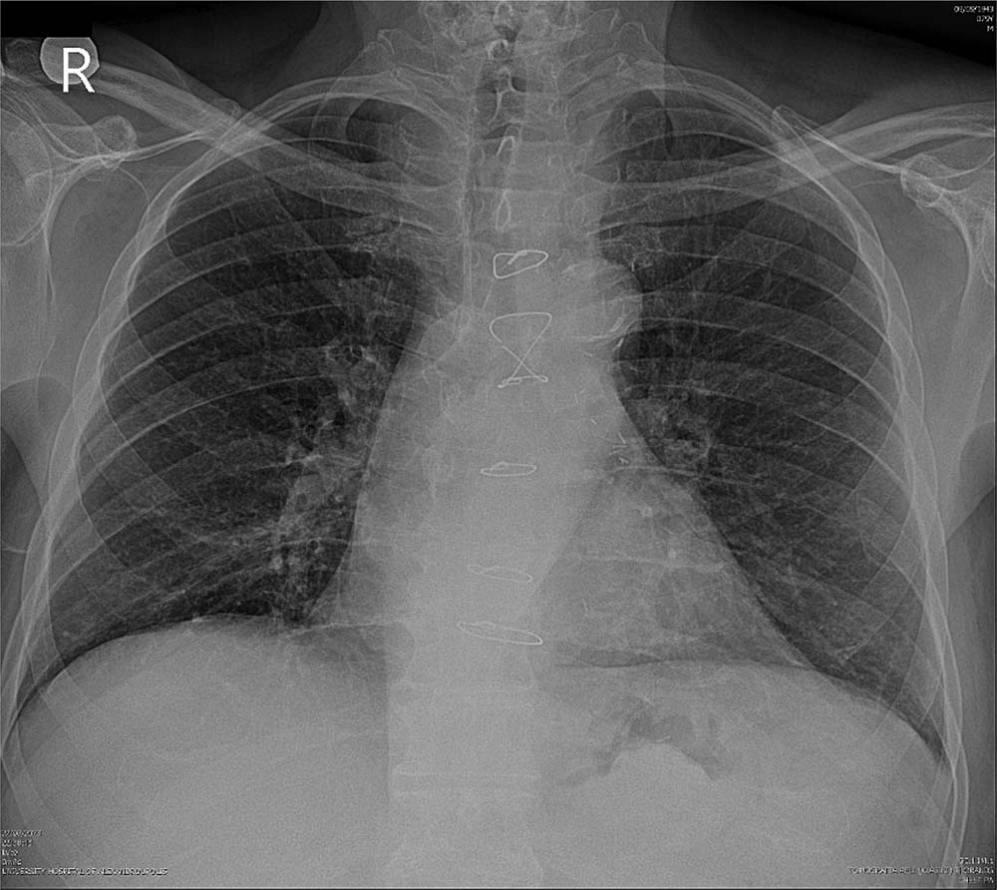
Preoperative chest X-ray.
